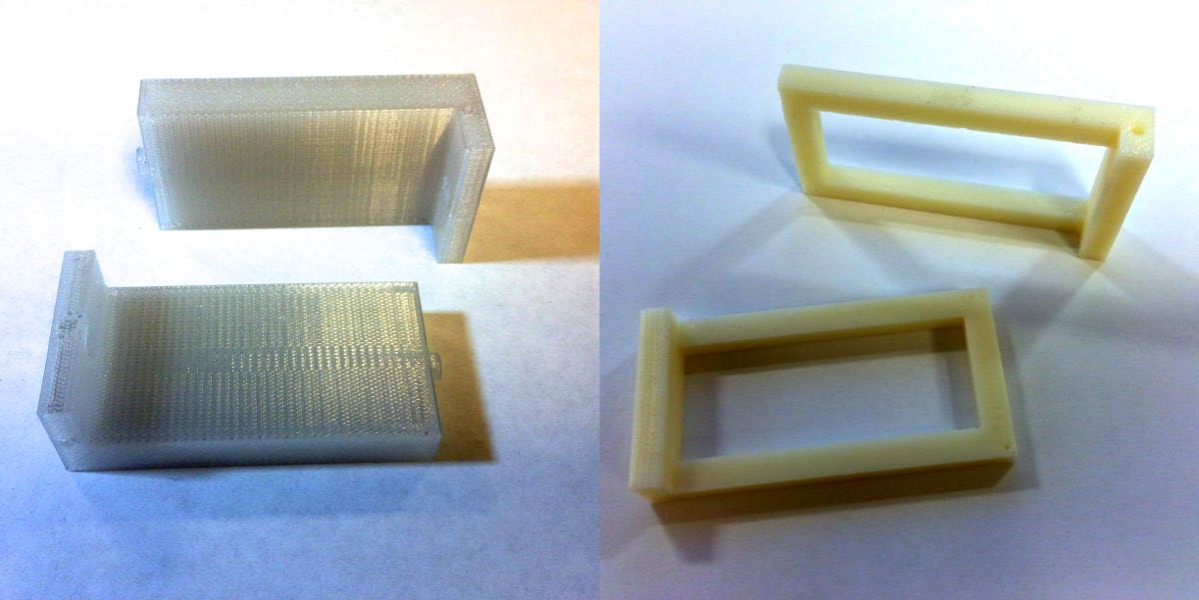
A set of testing apparati were designed and 3D printed for this study to measure the depth dose curve for ${}^{192}\text{Ir}$. The apparati held a piece of radiochromic film and an endobronchial brachytherapy catheter. The left picture shows the testing apparatus printed in PC‐ISO, and the picture on the right shows control apparatus used to suspend a piece of radiochromic film in water. The apparati were scanned in a helical CT to compute the Hounsfield unit distribution.
